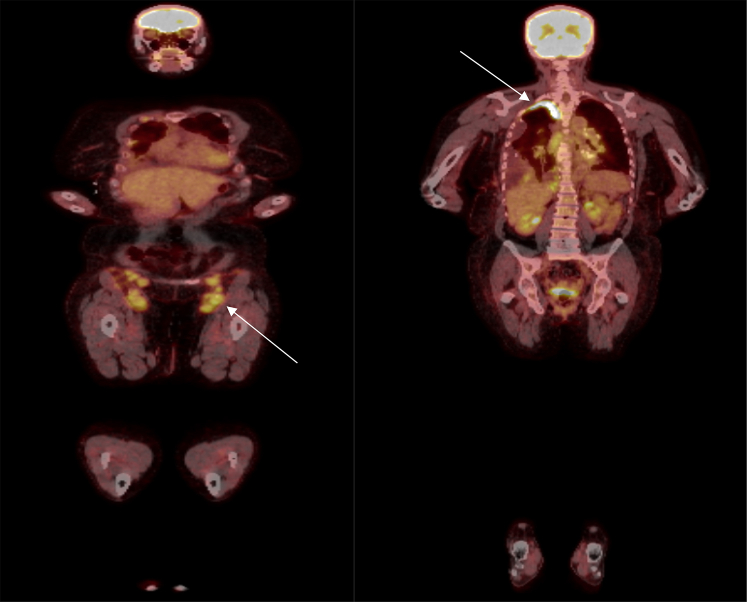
PET-CT coronal windows showing tracer uptake in the inguinal nodes bilaterally (white arrow) suggesting active disease tracer along with uptake in the pleura of the right hemithorax (white arrow) representing reactive granulomatosis related to talc pleurodesis.
